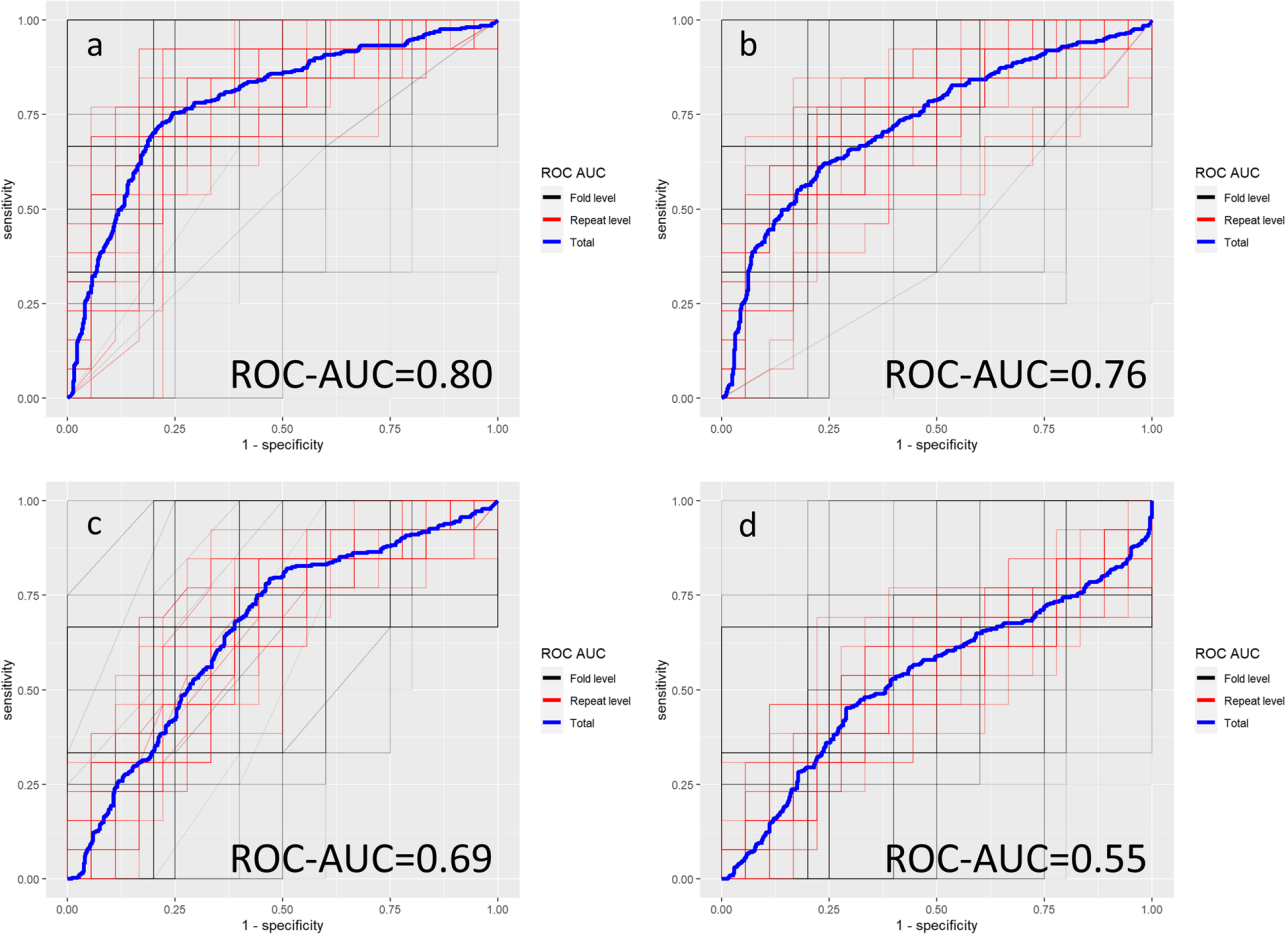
Receiver operating characteristic (ROC) curves for the four final models based upon the three most frequently LASSO-selected features (= most predictive features for cytogenetic risk classification). a In the entire multiparametric MRI protocol including all sequences (conventional anatomical whole-body MRI + spinal dynamic contrast-enhanced MRI + spinal diffusion-weighted imaging). b In the conventional anatomical whole-body MRI sequence only. c In the spinal dynamic contrast-enhanced MRI sequence only. d In the spinal diffusion-weighted MRI sequence only. Overall statistical performance is expressed by the ROC-AUC (receiver operating characteristic area-under-the-curve)
